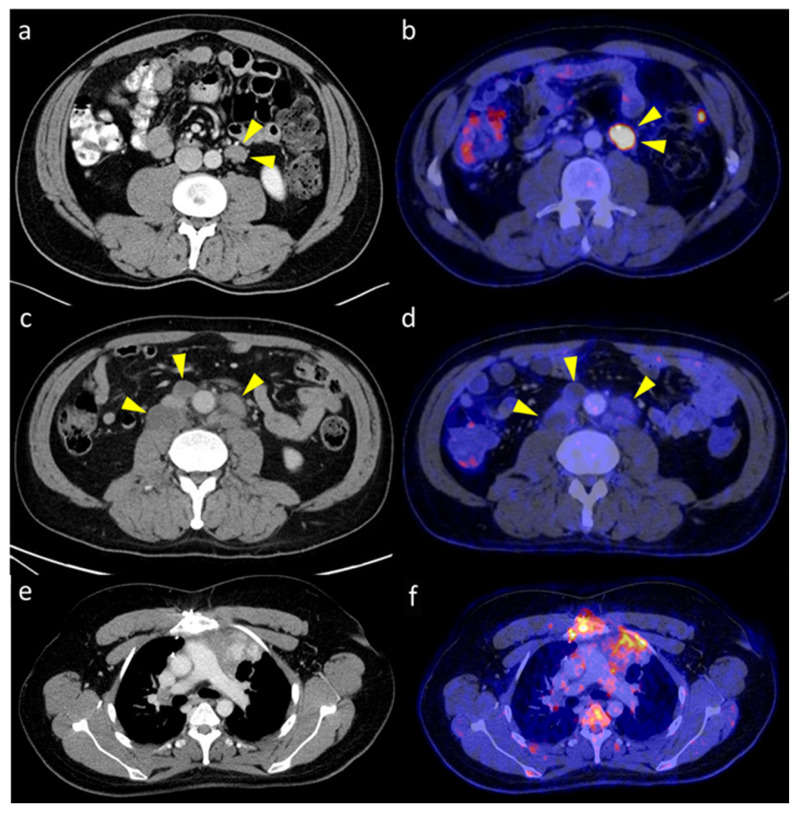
[18F]FDG PET/CT and additional CT of patients with GCTs: (a,b) A patient with testicular seminoma and suspicious para-aortal lymph node on CT showing increased FDG uptake on PET/CT, leading to upstaging; (c,d) a patient with testicular seminoma and suspicious retroperitoneal lymph nodes on CT that appears without FDG uptake on PET/CT, resulting in downstaging; (e,f) a patient with mediastinal seminoma and residual mediastinal mass on CT that was found to be recurrent on PET/CT.
